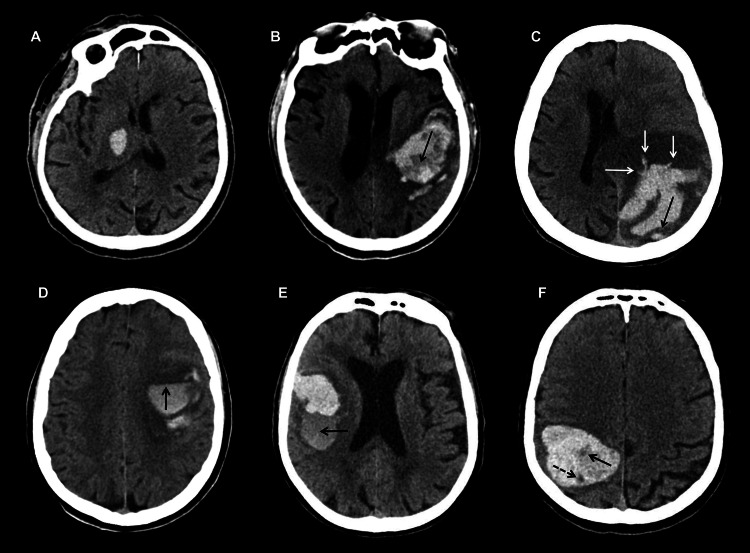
CT image showing intracerebral bleed. The lesion is causing a mass effect in the form of effacement of the right lateral ventricle adjacent sulcogyral spaces and a midline shift of 5.6 mm to left. There is an ill-defined area of hypodensity in the right frontal region. Mucosal thickening was noted in bilateral maxillary and ethmoid sinuses. Deviated nasal septum with a nasal spur to the right.
